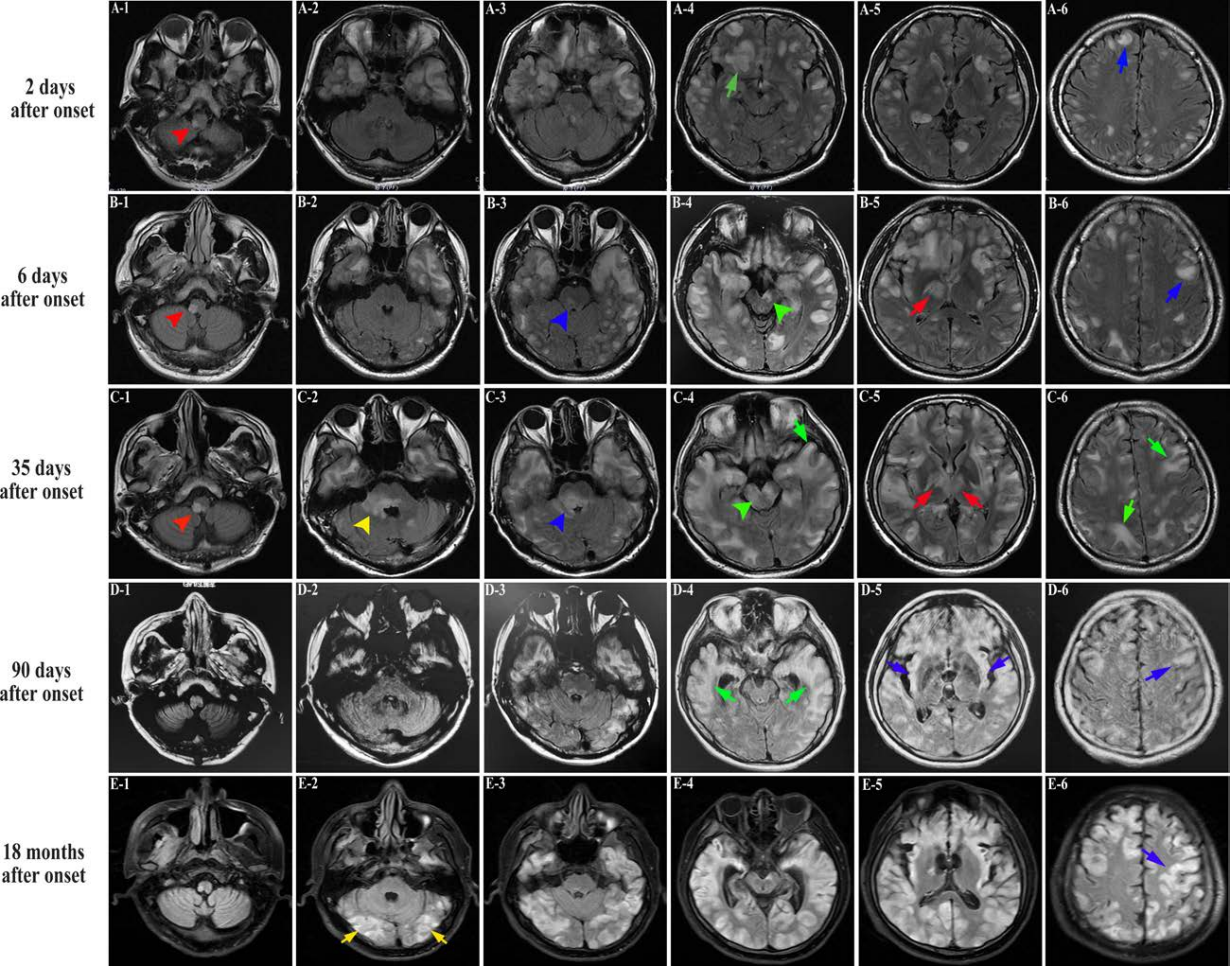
Axial T2-weighted FLAIR images showing lesions in each section at different times after onset. Second day (A1‐A6); 6th day (B1‐B6); 35th day (C1‐C6); 90th day (D1‐D6); 18th months (E1‐E6); confluent widespread hyperintensities of T2-FLAIR sections demonstrate the involvement of medulla (red arrowhead in A1, B1, C1) pons (blue arrowhead in B3, C3) midbrain (green arrowhead in B4, C4), medipeduncle (yellow arrowhead in C2), cerebellar hemisphere (yellow arrow in E2), thalami (red arrow in B5, C5) cortex (blue arrow in A6, B6, D5, D6, E6) subcortex and white matter (green arrow in A4, C4,C6, D4). MR images on day 6 and 35 reveal marked increased number and mass effect of the lesions.
